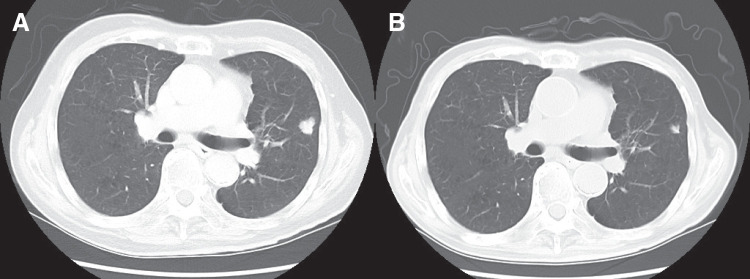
(A) CT scan on a day in August 2024, revealed lung metastases. (B) A reduction in the size of the metastatic lesions was observed after chemotherapy.
